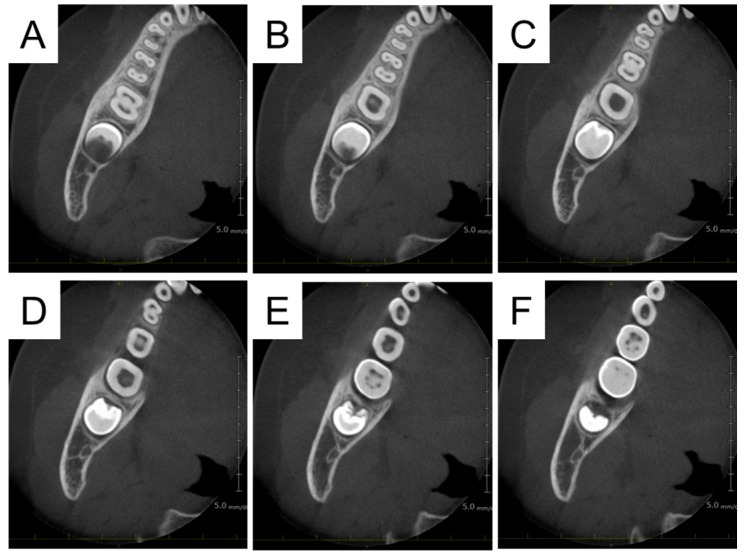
CBCT images at the age of 7 years and 11 months. The bone defect on the buccal side of the first molar had disappeared, and the continuity of the alveolar bone was confirmed, suggesting that the dentoalveolar abscess had completely healed (A–F).
